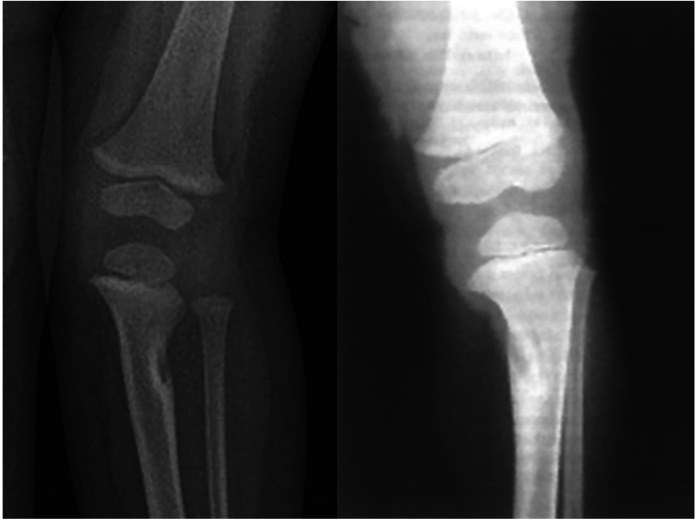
Two examples of FFCD; on the left, we can note an unusual valgus deformity in the left tibia characterised by a lateral defect surrounded by cortical condensation in its proximal third. On the right side, AP radiograph revealed a well-defined lucent defect in the medial cortex of the proximal tibia with varus deformity.
